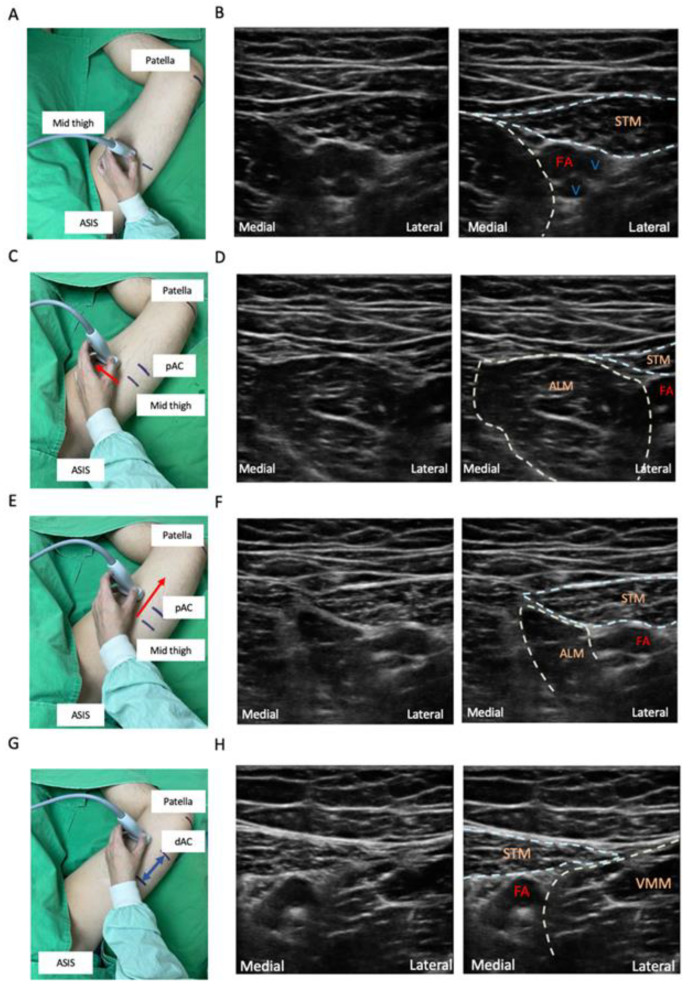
Ultrasound identification of the adductor canal. (A) Mark the mid-thigh location midway between the ASIS and base of the patella. Place the probe transversely to obtain short axis view. (B) Identify the femoral vessels. Distinguish the artery by compression sign. (C) Slide the probe medially (red arrow) to visualize (D) the medial borders of the sartorius and adductor longus muscle. (E) Slide the probe caudally (red arrow) along the medial borders of the sartorius muscle and the adductor longus muscle to visualize (F) the point of the start of the adductor canal, intersection of the medial borders of sartorius muscle and adductor longus muscle. (G) Adjust the probe to make the neurovascular bundle on the medial side of the ultrasound screen. Then, slide the probe caudally until (H) the femoral artery goes deep to the adductor hiatus. ASIS, anterior superior iliac spine; pAC, proximal adductor canal; dAC, distal adductor canal; FA, femoral artery; V, femoral vein; STM, sartorius muscle; ALM, adductor longus muscle; VMM, vastus medialis muscle.
